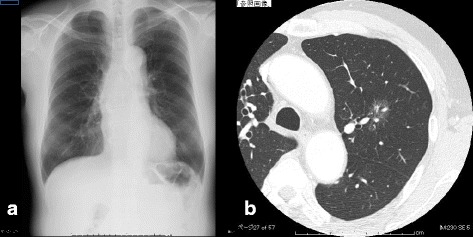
Preoperative imaging. a Preoperative chest X-ray reveals no abnormal sign. b Preoperative CT reveals a 30-mm ground glass nodule left upper lobe
